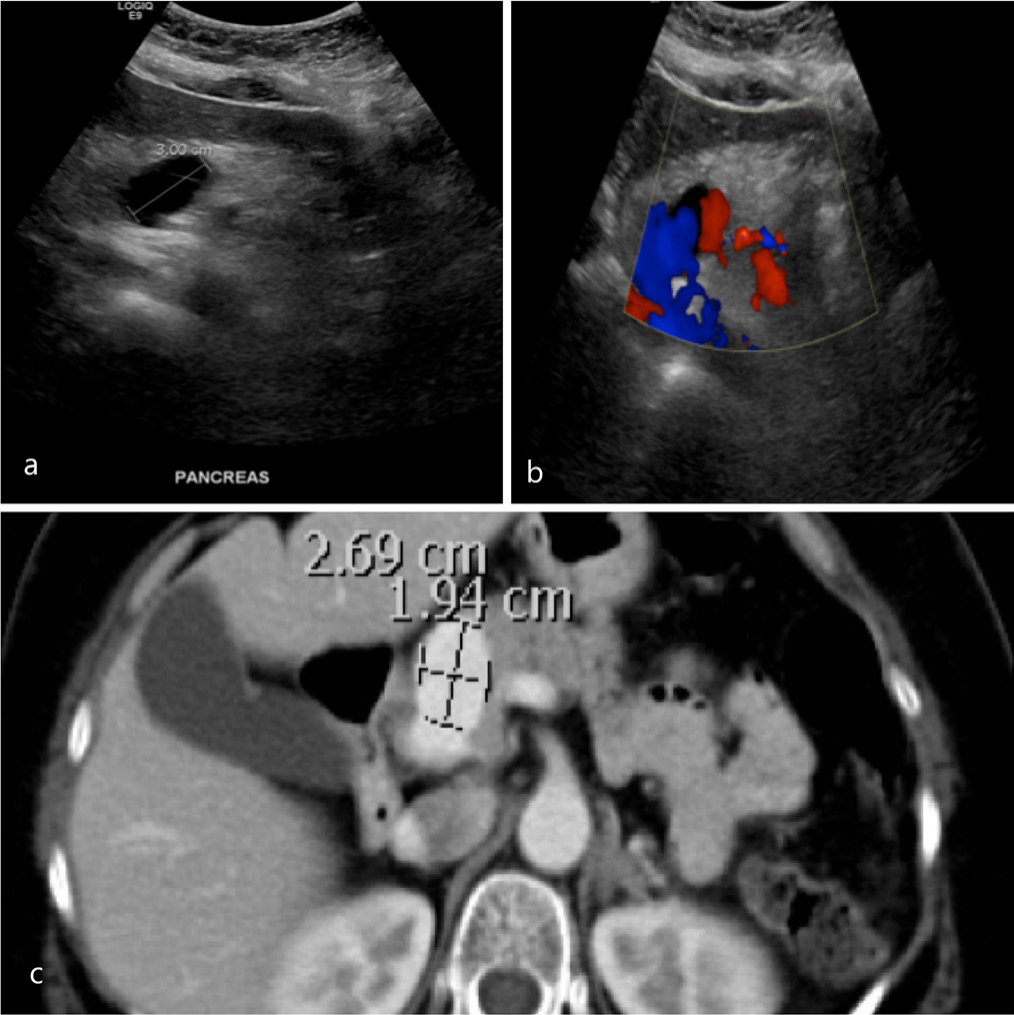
Greyscale (a) and colour Doppler ultrasound (b) of the abdomen demonstrated an anechoic cystic lesion measuring 3 cm near the head of the pancreas with an internal vascularity. (c) A contrast-enhanced CT scan in portal venous phase showed a main portal vein focal dilatation measuring 2.69 × 1.94 cm.
